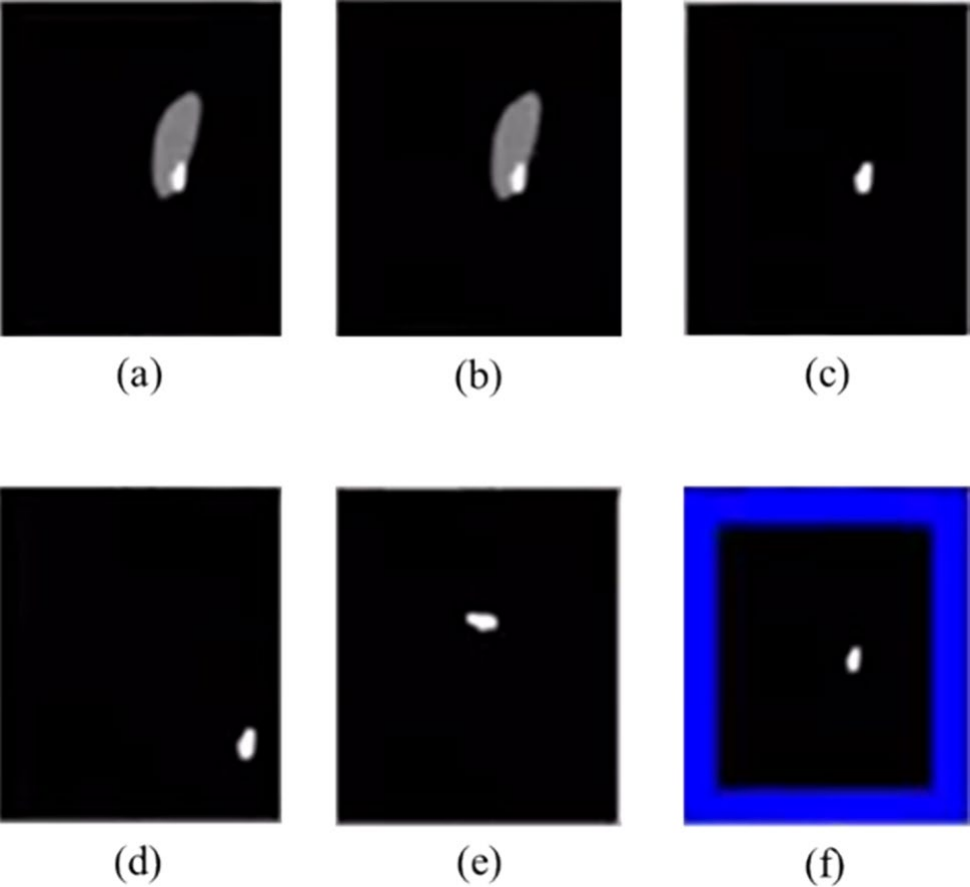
Experimental image outcomes. a Input, b pre-processed, c segmented, d translation, e rotation, and f padding augmented CT image
